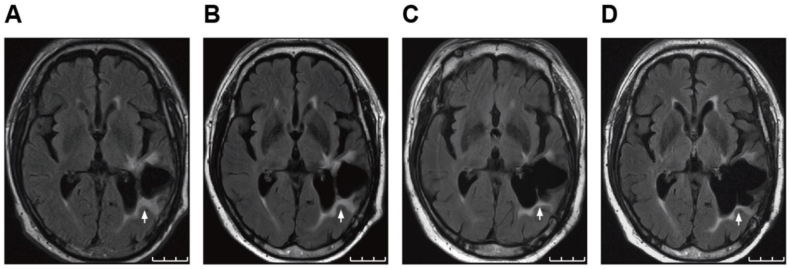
T2-weighted FLAIR MRI following bevacizumab treatment. Images were acquired after two cycles (A), after six cycles (B), at 20 months post-treatment (C), and at 44 months post-treatment (D). Arrows indicate areas of high signal intensity on T2-weighted FLAIR MRI, showing a gradual decrease during and after bevacizumab treatment.
